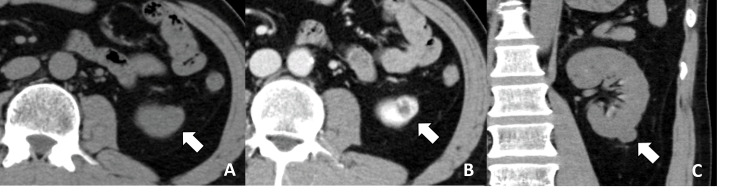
CT images of a 50-year-old man with clear cell RCC A: unenhanced image; B: enhanced image; C: unenhanced coronal image On axial UE-CT, a 1.8-cm heterogeneous isodense mass with unclear margins is seen in the left kidney (arrows). The mass protrudes slightly outside the renal cortex. When multiplanar reconstruction is used, the mass can be detected in the lower pole of the kidney by visualizing the protrusion of the mass on the coronal image RCC: renal cell carcinoma; UE-CT: unenhanced computed tomography
